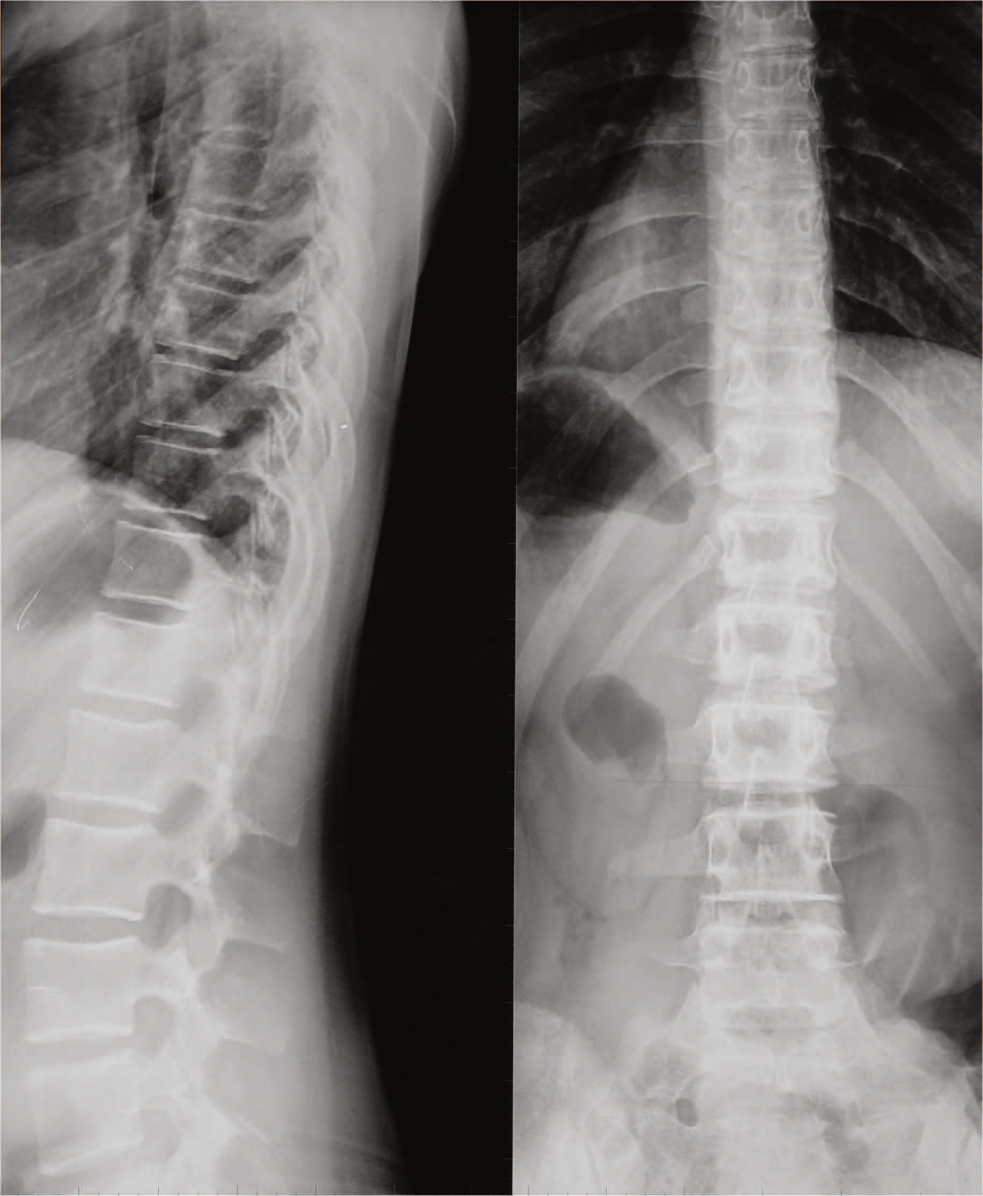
Standing (brace not worn) posterior-anterior and lateral radiographs 24 months after the initial application of the brace (Visit 3). The vertebral rotation has significantly improved in most of the affected vertebrae.
